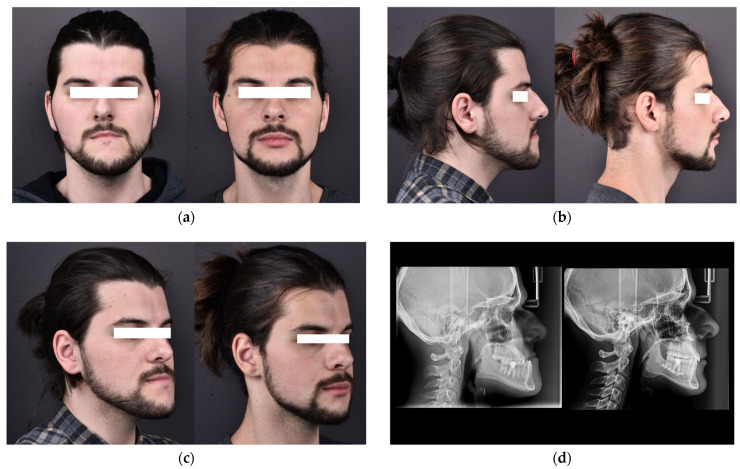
Pre- and postoperative views of the patient after orthognathic surgery (maxillomandibular advancement, mandibular setback, and counterclockwise rotation). (a) Frontal view. (b) Right profile. (c) Left semi-profile. (d) Lateral cephalometric radiograph.
